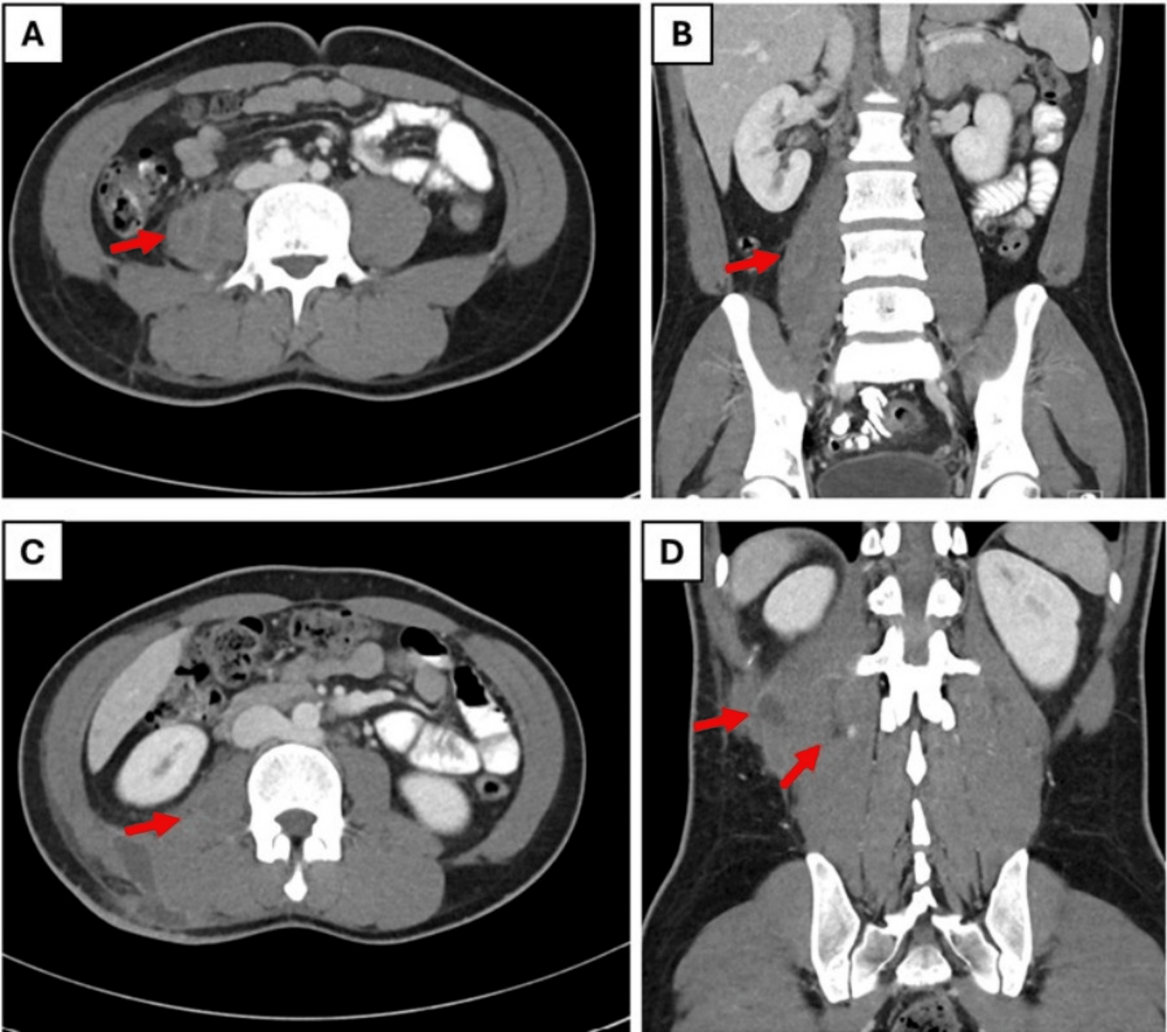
Third follow-up (one-month interval) selected axial (A, C) and coronal (B, D) contrast-enhanced CT images reveal continued regression of the previous right psoas and iliacus abscess, with resolution of associated muscle swelling A new multiloculated fluid collection is now identified along the right paraspinal muscle plane, suggesting possible extension or recurrence in a different myofascial compartment.
